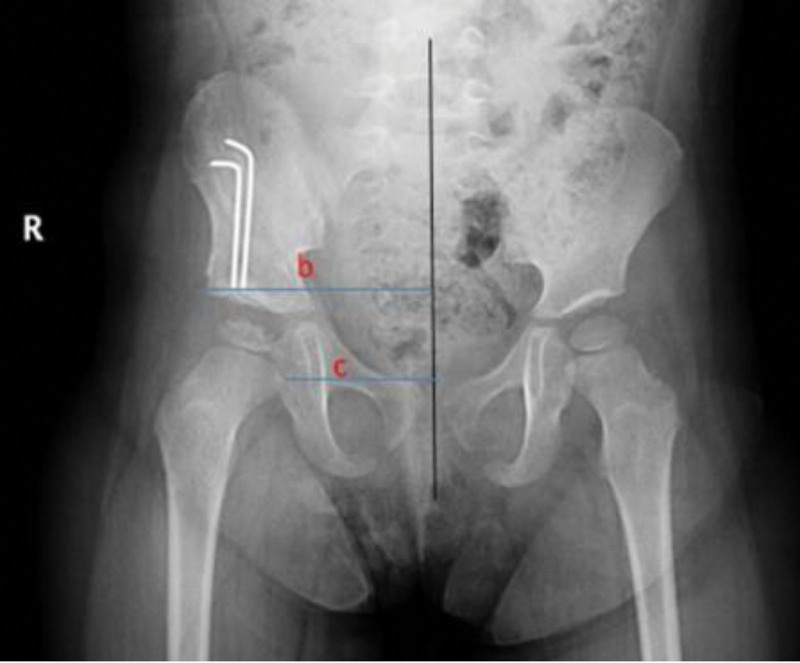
Teardrop figure measurements. Pelvic radiograph of a 25-month-old girl who underwent salter innominate osteotomy of both hips. TDW (W: TDW) and TDD (D: TDD) measurements are shown on the right hip, while ATA (A: ATA) measurement is shown on the left hip. ATA = acetabular teardrop angle, TDD = femoral head teardrop distance, TDW = teardrop width.
